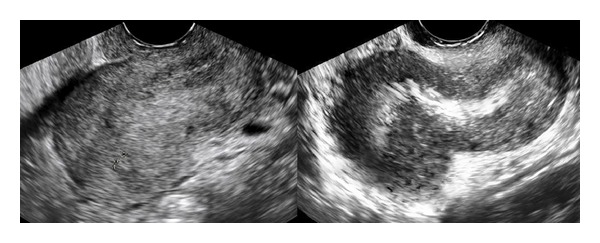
Ultrasound image of question mark sign.
